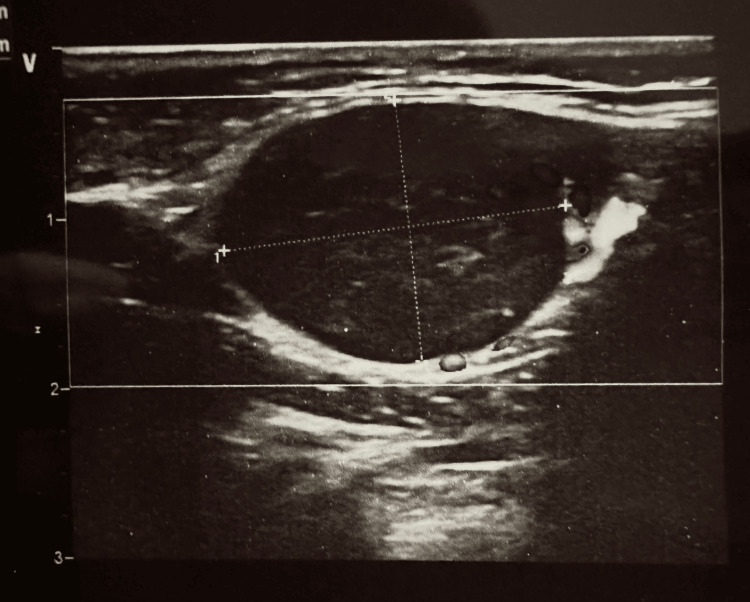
Ultrasonography revealed bilaterally enlarged cervical lymph nodes, with the largest up to 2.4x0.8 cm in size and a length-to-width ratio of less than 0.5
